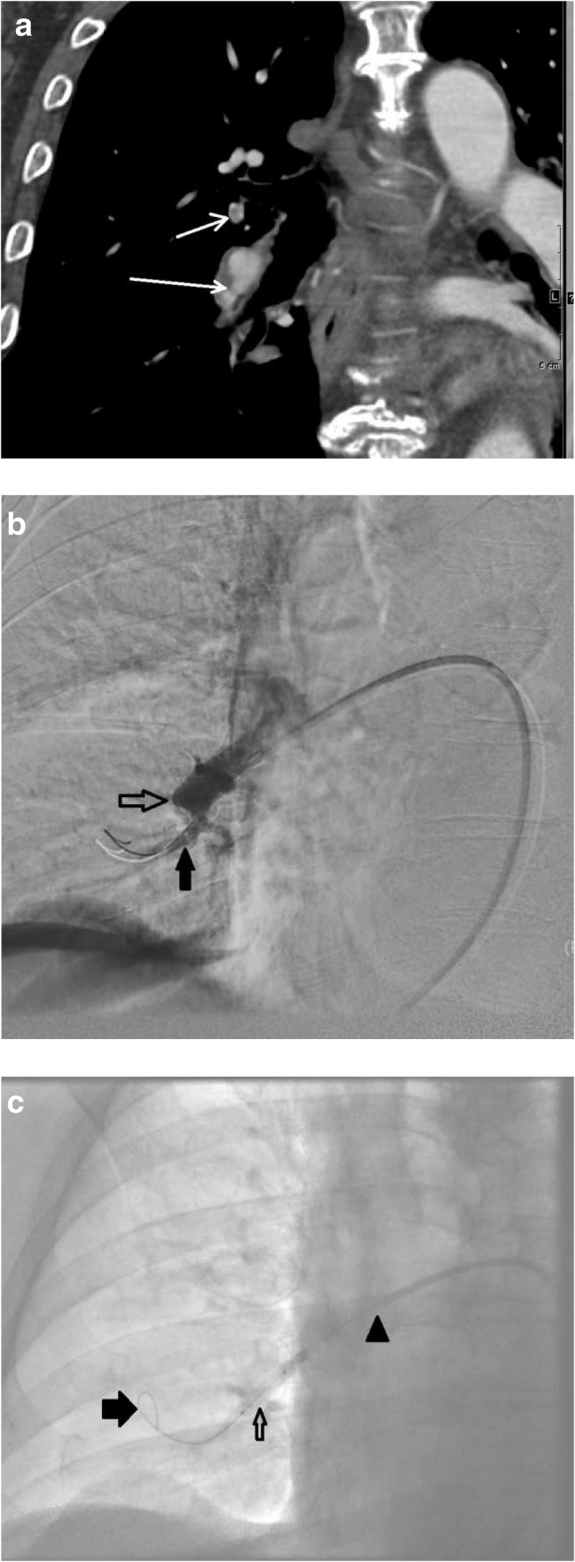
Imaging and treatment in a patient with CTEPH. An 82-year-old male patient, nonsurgical candidate, with central-type and distal-type CTEPH. a CTA demonstrating webs and bands (white arrows). b Region of stenosis (solid black arrow) and occlusion (open black arrow) due to webs and bands. c Fluoroscopic image of balloon pulmonary angioplasty. A 4 mm balloon (open black arrow), passed through a 7 Fr 90 cm Brite Tip sheath (Cordis, Milpitas, CA, USA, arrow head), over a V18 (Boston Scientific, Malborough, MA) working wire (solid black arrow). d Pulmonary angiogram demonstrates revascularized segmental pulmonary arteries (black arrows)
